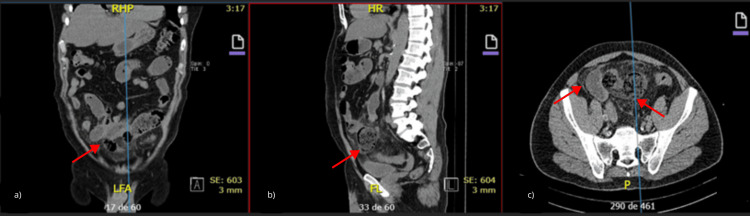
Abdominal CT demonstrating transition point and proximal dilatation Abdominal computed tomography (CT) in (a) coronal, (b) sagittal, and (c) axial sections demonstrating a pelvic inflammatory process with mesenteric fat stranding, a dilated ileal loop with fecaloid content at the transition point, dilated proximal jejunal loops with fluid, and free fluid in the pelvis (red arrows).
